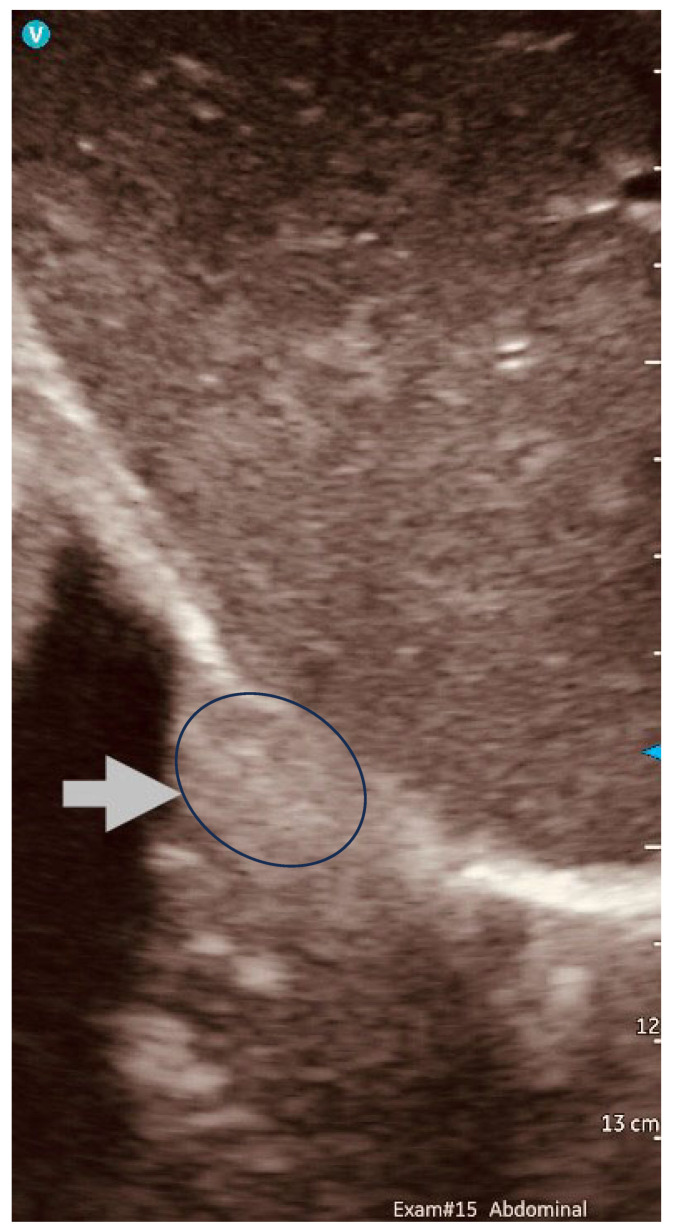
Introspective ultrasound scan image using a convex probe in open surgery to show a deep liver subcapsular deposit (arrow indicating deep liver deposit (circled)) (V on top-left is the orientation marker for GE Vuson indicating the ventral direction).
